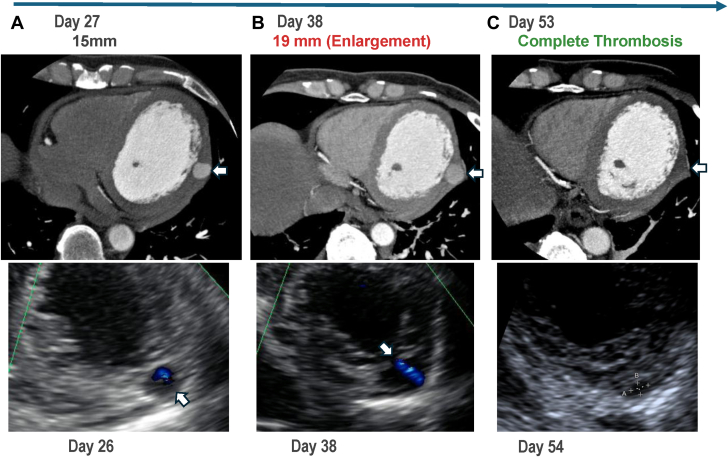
Serial Computed Tomography and Echocardiographic Imaging Demonstrating Left Ventricular Pseudoaneurysm Evolution Top row: Computed tomography images. Bottom row: echocardiography with color Doppler. (A) Day 26–27: pseudoaneurysm detected (15 mm on CT). (B) Day 38: enlargement (19 mm on CT). (C) Day 53–54: complete thrombosis. Arrows indicate the pseudoaneurysm on computed tomography and the corresponding echocardiographic findings.
